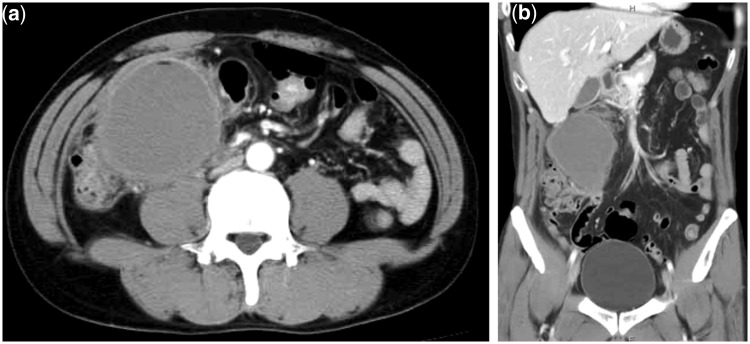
A contrast medium-enhanced computed tomography showing a large, unilocular cystic mass in the right retroperitoneal space: (a) axial view; (b) coronal view.
